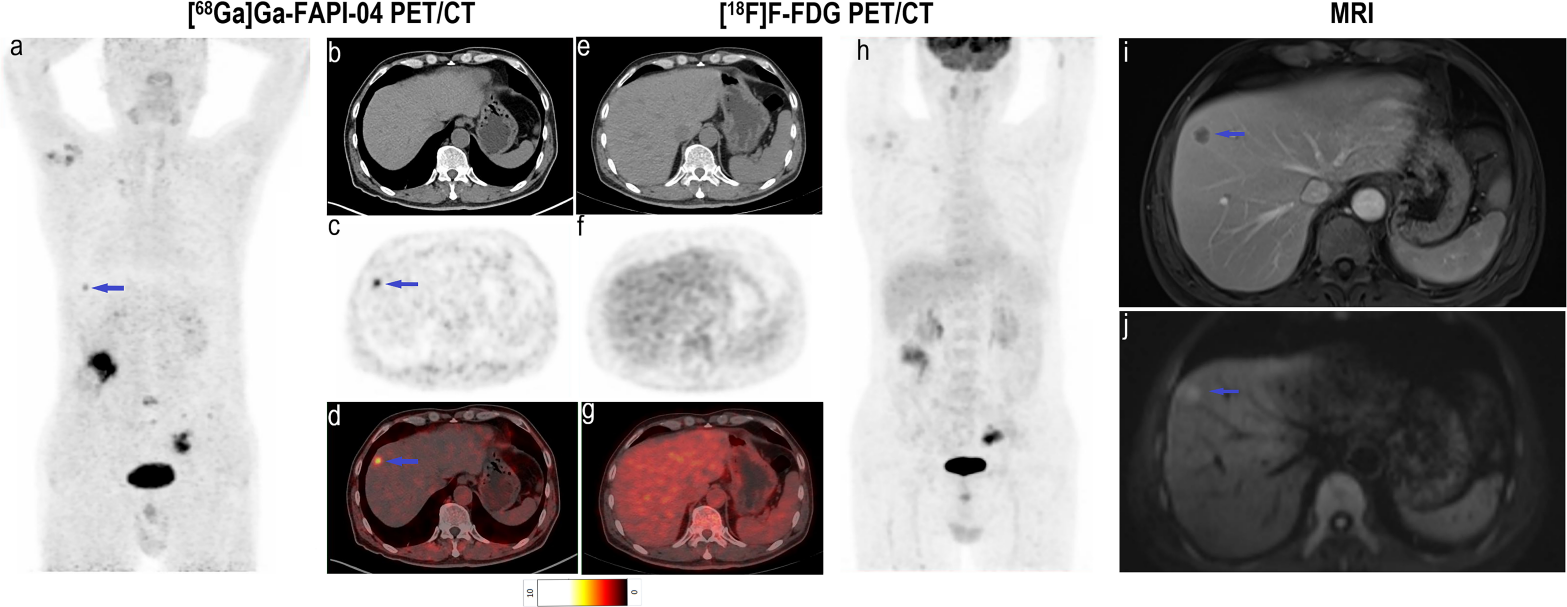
Images of a 63-year-old man with colon cancer for staging. (A) Mean intensity projection images of [^68^Ga]Ga-FAPI-04 PET/CT scans. (B–D) Axial CT, PET and fused images of [^68^Ga]Ga-FAPI-04 PET/CT scan. (E–G) Axial CT, PET and fused images of [^18^F]F-FDG PET/CT scan. (H) Mean intensity projection images of [^18^F]F-FDG PET/CT scan. (I, F) Images of contrast-enhanced MRI, T2WI (delay phase) and DWI (b = 1000). The suspicious metastatic lesion in the right liver lobe is clearly visible in [^68^Ga]Ga-FAPI-04 PET/CT images (blue arrows in A–D; SUV_max_ 5.0) but absent in [^18^F]F-FDG PET/CT images. Its presence was confirmed by contrast-enhanced MRI (blue arrows in I, J).
